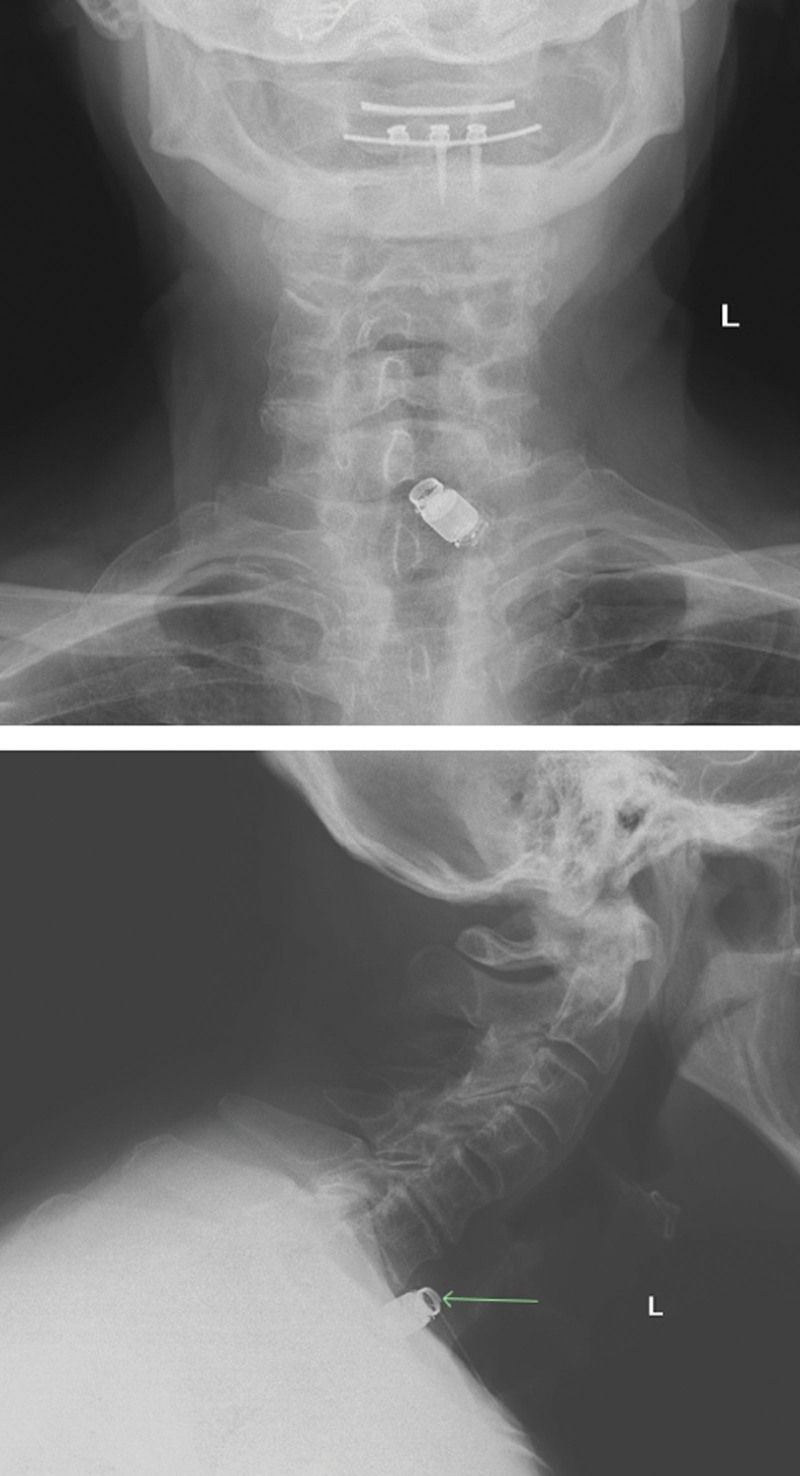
Soft tissue neck x-rays of capsule endoscopy retention in the neck.
